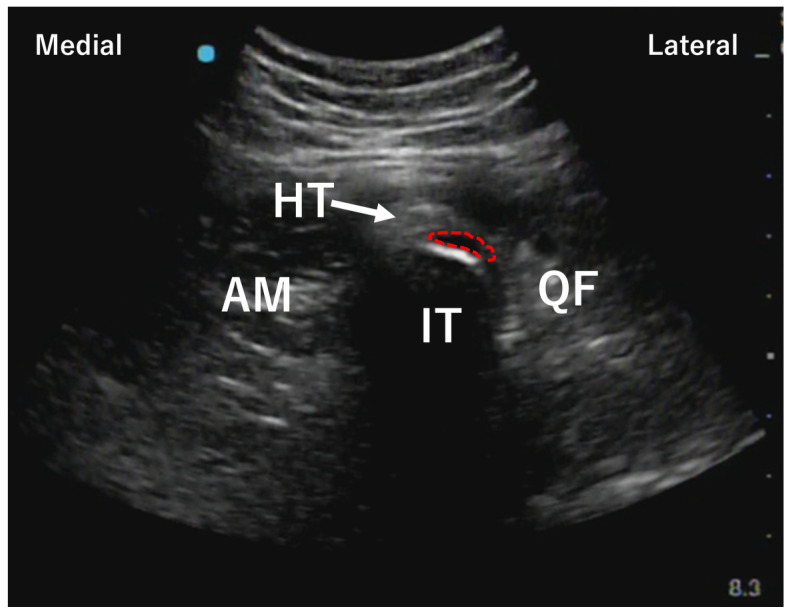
Ultrasound image of the ischial tuberosity showing the proximal attachment of the hamstring tendon. A short-axis ultrasound image at the ischial tuberosity demonstrated hypoechoic thickening of the proximal semimembranosus tendon. The probe was positioned in a transverse (short-axis) orientation, allowing visualization of the tendon cross-section and adjacent structures. A mildly hypoechoic (Red dot line) area is visible at the enthesis, without evidence of tendon thickening or tear. IT: ischial tuberosity; HT: hamstring tendon; AM: adductor magnus; QF: quadratus femoris.
